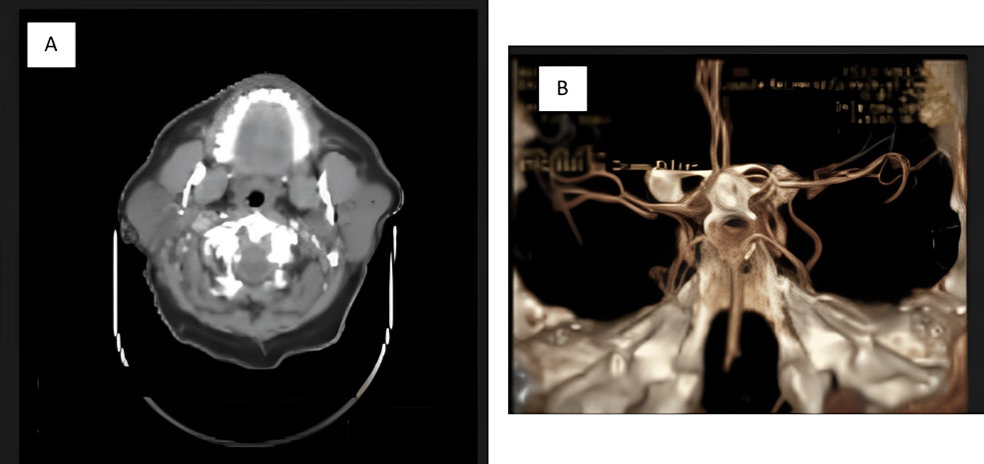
CT head and neck angiogram with and without intravenous contrast showing a normal brain with no abnormalities (A) and all large extra and intracranial vessels that are patent without evidence of aneurysm formation or beading of the distal intracranial vessels (B) CT: computed tomography
